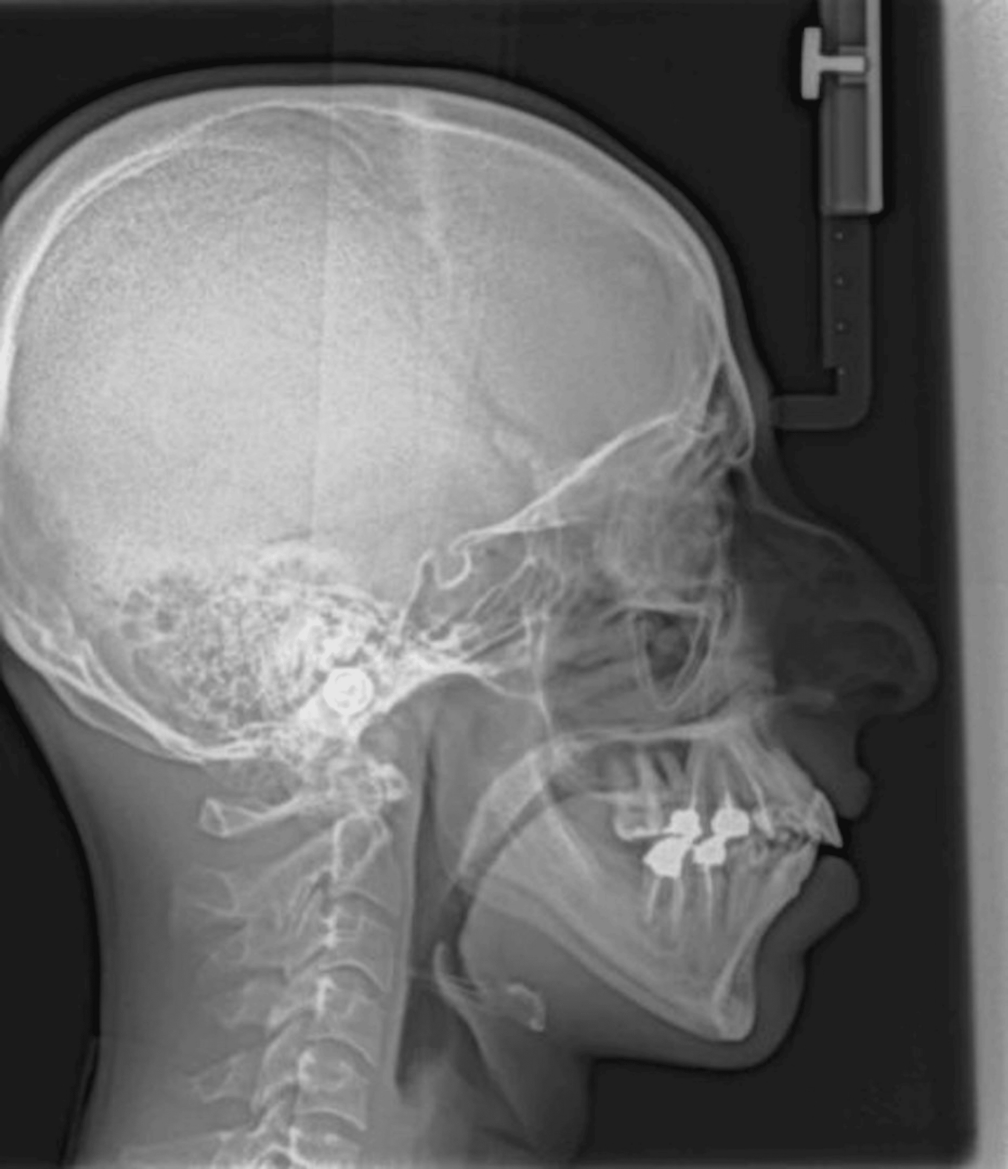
Cephalometry radiograph of male patient in Figure 1 with bimaxillary proclination.
